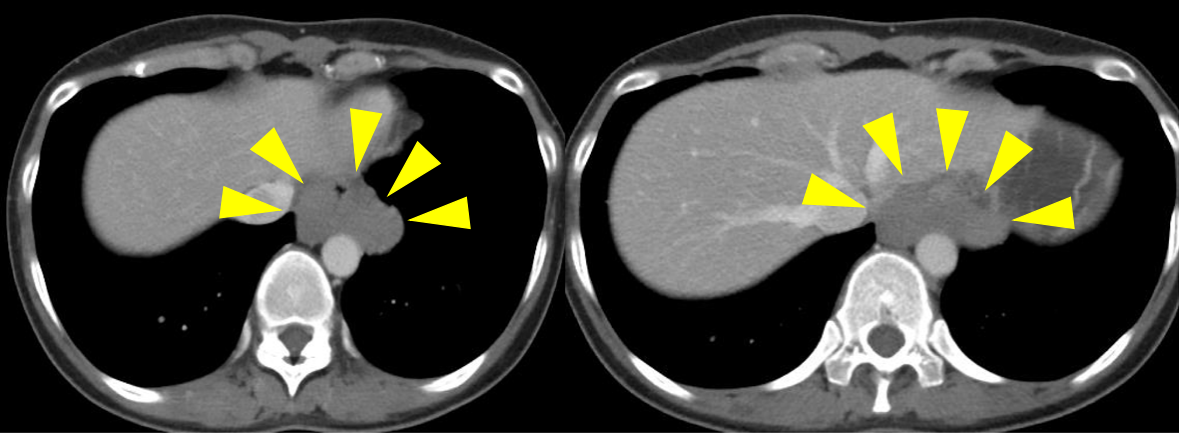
Computed tomographic images. A tumor surrounding the esophagus is seen at the lower thoracic esophagus measuring > 60 mm (major axis) with a complicated shape
